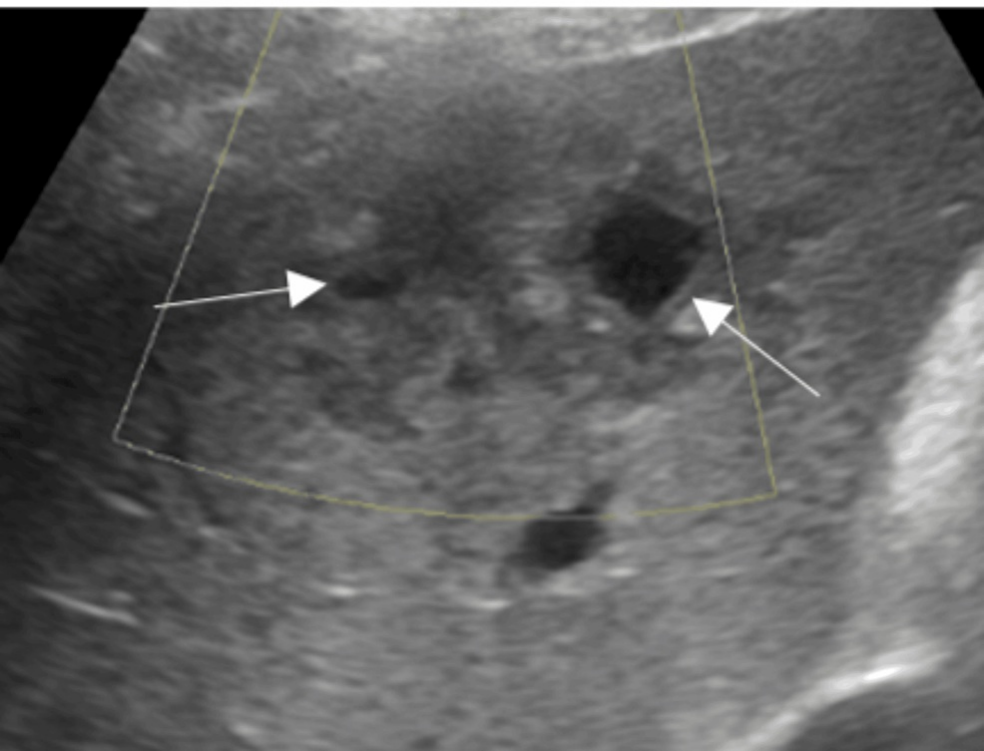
Ultrasound of the abdomen showing liver abscess displaying a regression in size.
